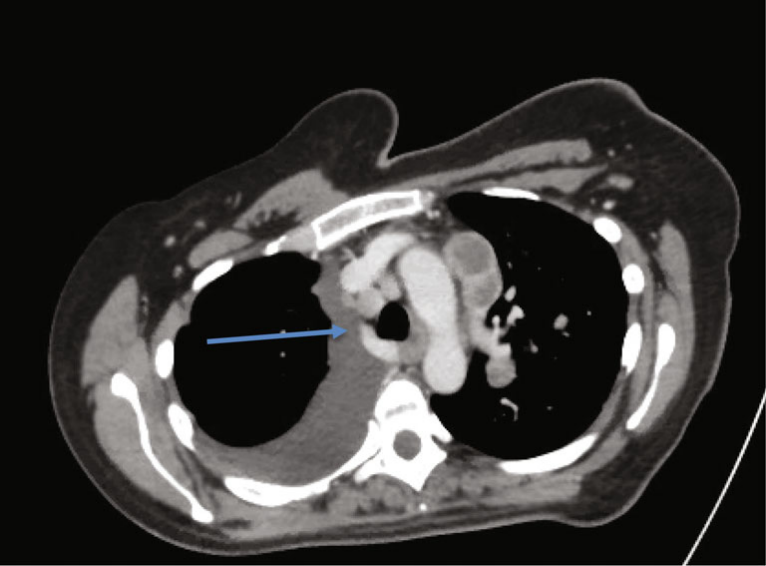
CT chest showing involvement of the right lower paratracheal lymphnodes (Station 4R).
